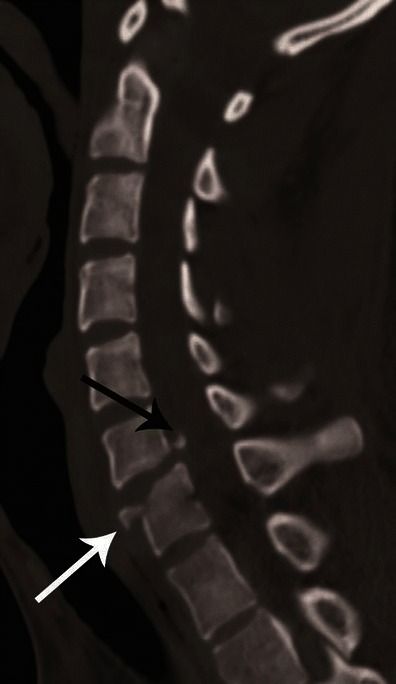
Avulsion fracture in hyperextension dislocation. Sagittal CT image shows an avulsion fracture at the anterosuperior end plate of C7 (*white arrow*), with the transverse diameter wider than the vertical diameter. Avulsion of the posteroinferior corner of the C6 vertebral body is also seen (*black arrow*), which suggests avulsion of the posterior annulus or PLL
